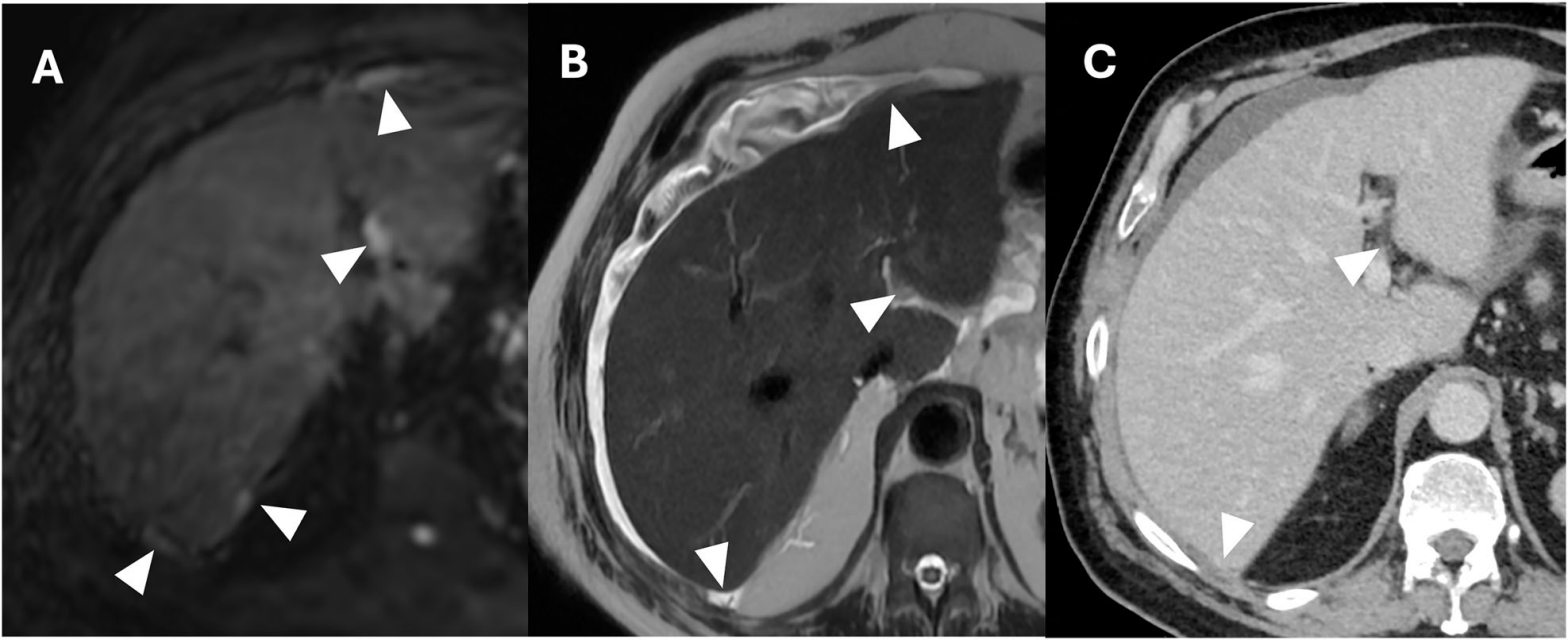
Pancreatic cancer with peritoneal carcinomatosis in a 64-year-old woman. MRI DWI (b = 800 mm^2^/s) (A) demonstrated four nodular implants in the right hypochondrium (arrowheads), of which only three were evident on T2w (arrowheads) (B) and two on CT portal-venous phase (arrowheads) (C)
